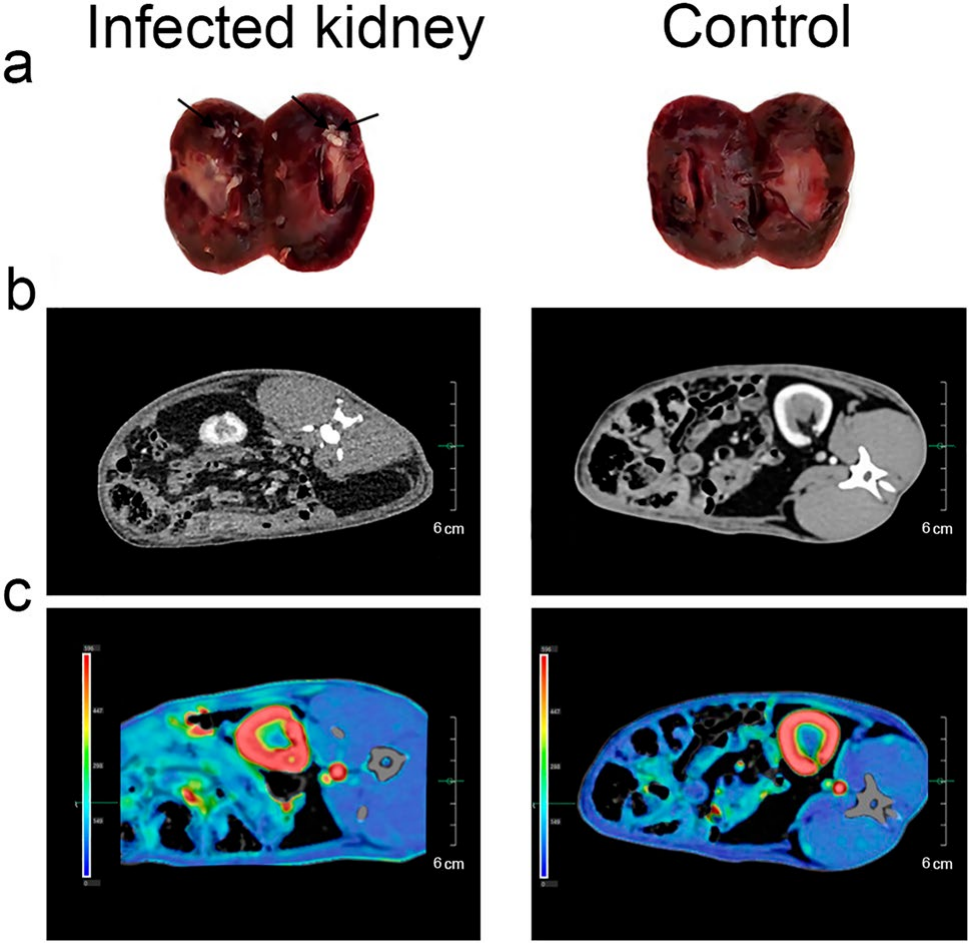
Assessment of the development of rTB. (a) Macrophotos of the cross section of the kidney. Black arrows show foci of TB development. Abdominal CT: (b) the zone of inflammation is seen as the hypovascular area in the cortical and medullar layer on the left and intact kidney on the right. (c) CT perfusion of the kidney: hypoperfusion region in the kidney that correlates with area of destruction (green colour) on the left and normal layer differentiation with no destruction areas on the right.
